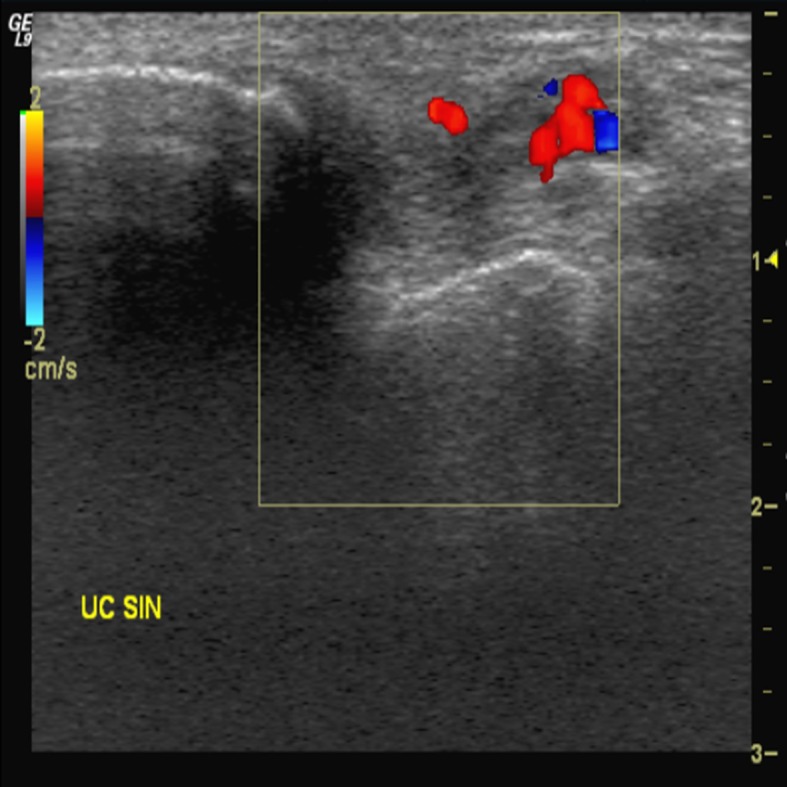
Vascularization in ulnocarpal joint – group 2: more Doppler signals in vessels, but on the surface that is smaller than the half of the complete size of synovia.
